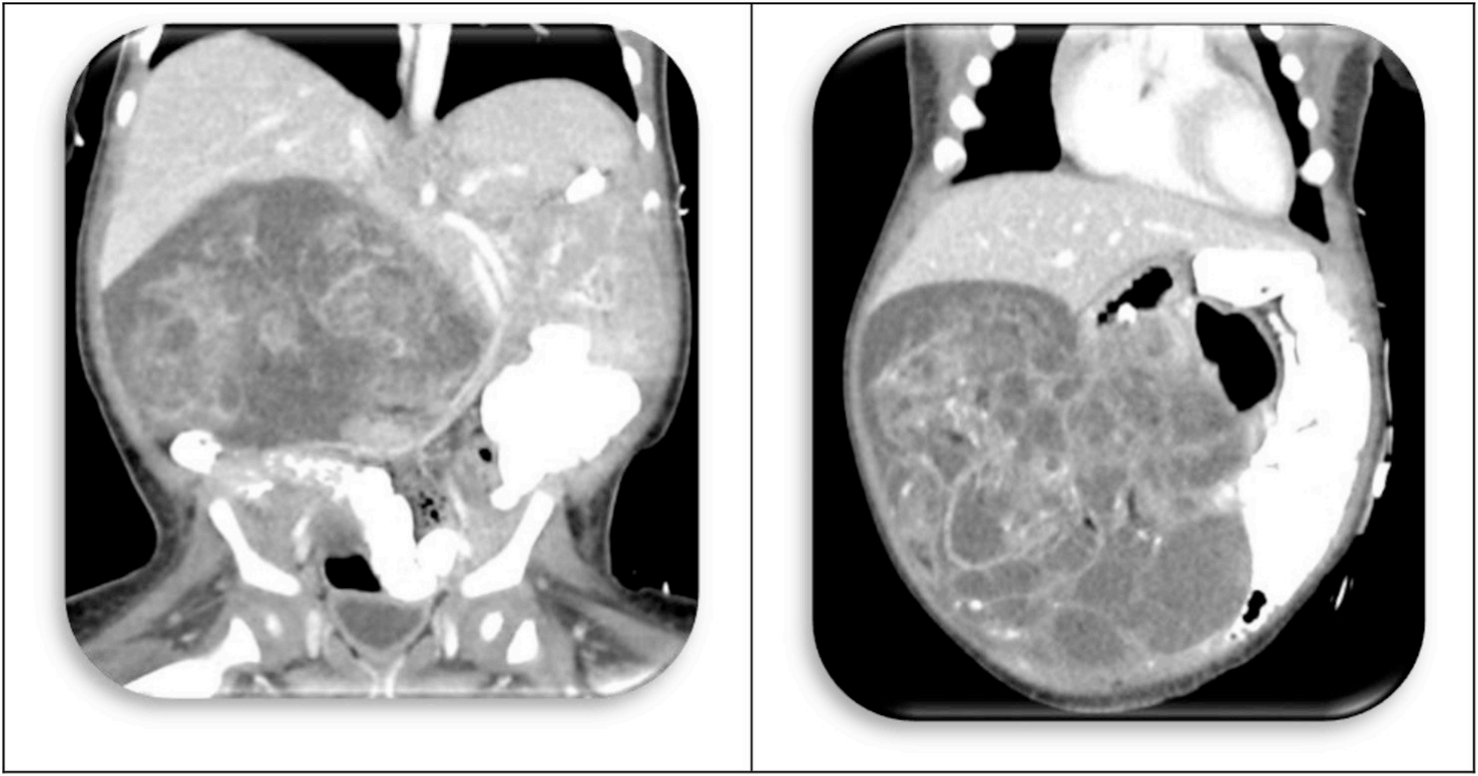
CT scan of Gastric Teratoma.
